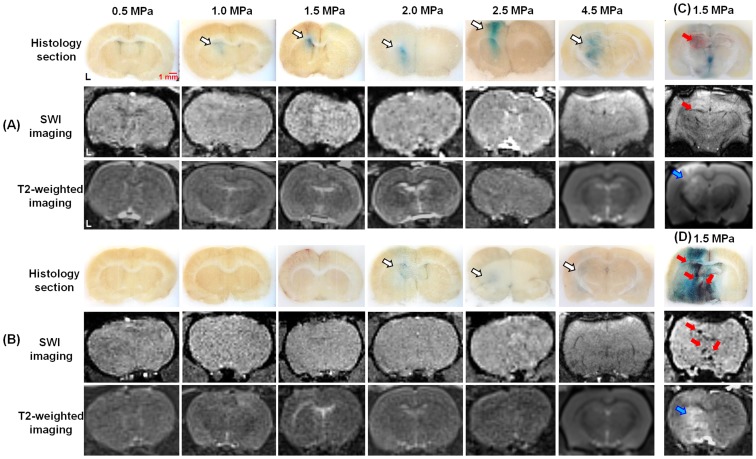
MRI results after FUS sonication. A: In-house submicron bubbles with 10-MHz FUS sonication. B: SonoVue with 10-MHz FUS sonication. C: In-house submicron bubbles with 1-MHz FUS sonication. D: SonoVue with 1-MHz FUS sonication. (white arrows: the BBB-opening region; red arrows: areas of erythrocyte extravasations; blue arrows: brain edema).
